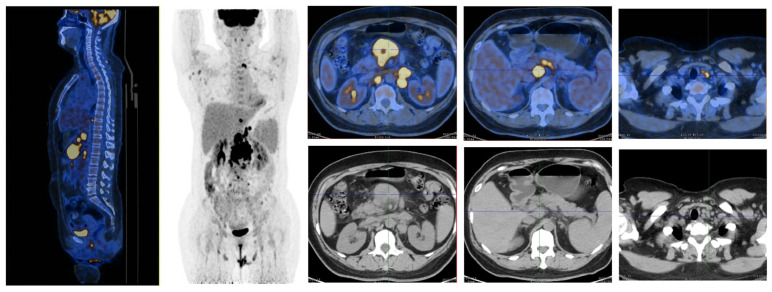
The positron emission tomography–computed tomography (PET/CT) images demonstrated extensive enlargement of the lymph nodes with increased fluorine-18-fluorodeoxyglucose (FDG) metabolism at initial diagnosis.
